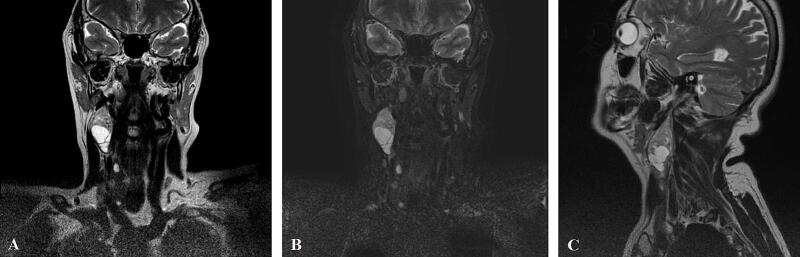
MRI scan indicating neoplasm of carotid sheath of the right side of neck. A- coronal plane T2 sequence, B- coronal plane T2 STIR sequence, C- sagittal plane T2 sequence.
